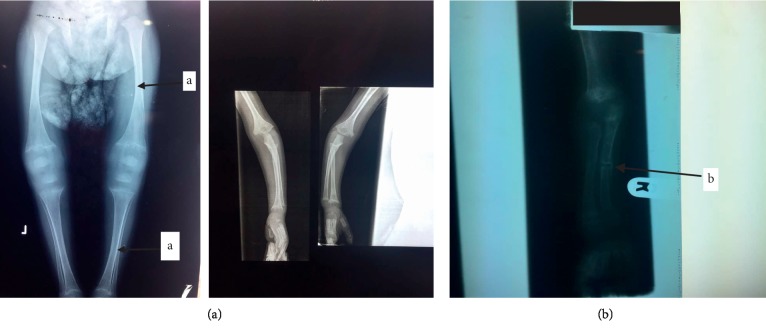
(a) Bowing of limbs with cortical thinning; (b) X-ray findings which were done at 4 years of age showed fracture of the midradius.
